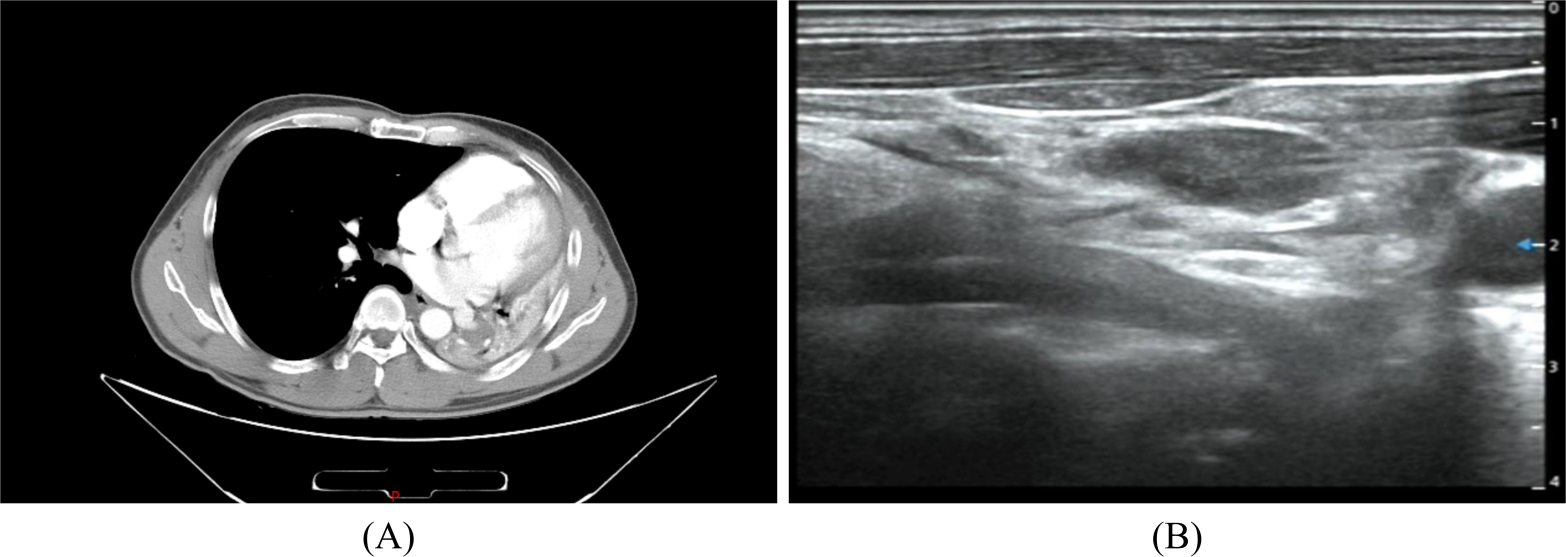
Chest CT (A) The left lung is reduced in volume and multiple nodules are seen in the lower left lung. Neck ultrasound (B) Multiple swollen lymph nodes in the neck.
